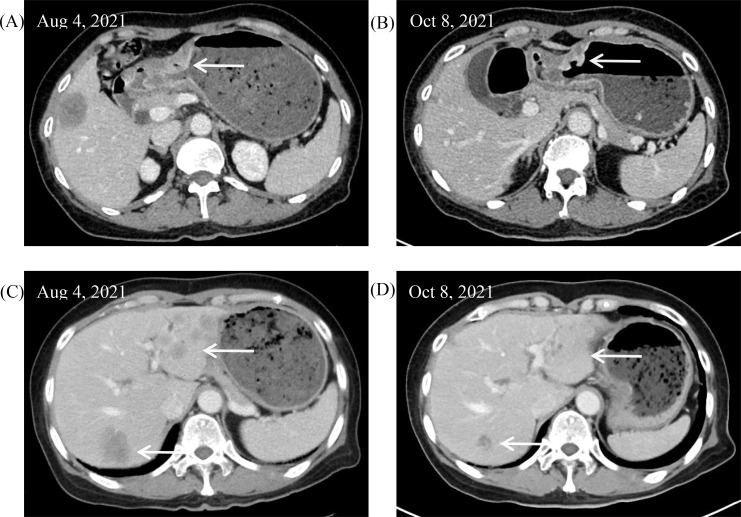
Follow-up CT scans confirming a partial response (PR) after two cycles of infigratinib. (A, B) Case 2 showing the gastric lesion in CT images before therapy and stable after therapy. (C, D) Case 2 showing marked liver metastases in CT images before therapy and a significant shrinkage after therapy.
